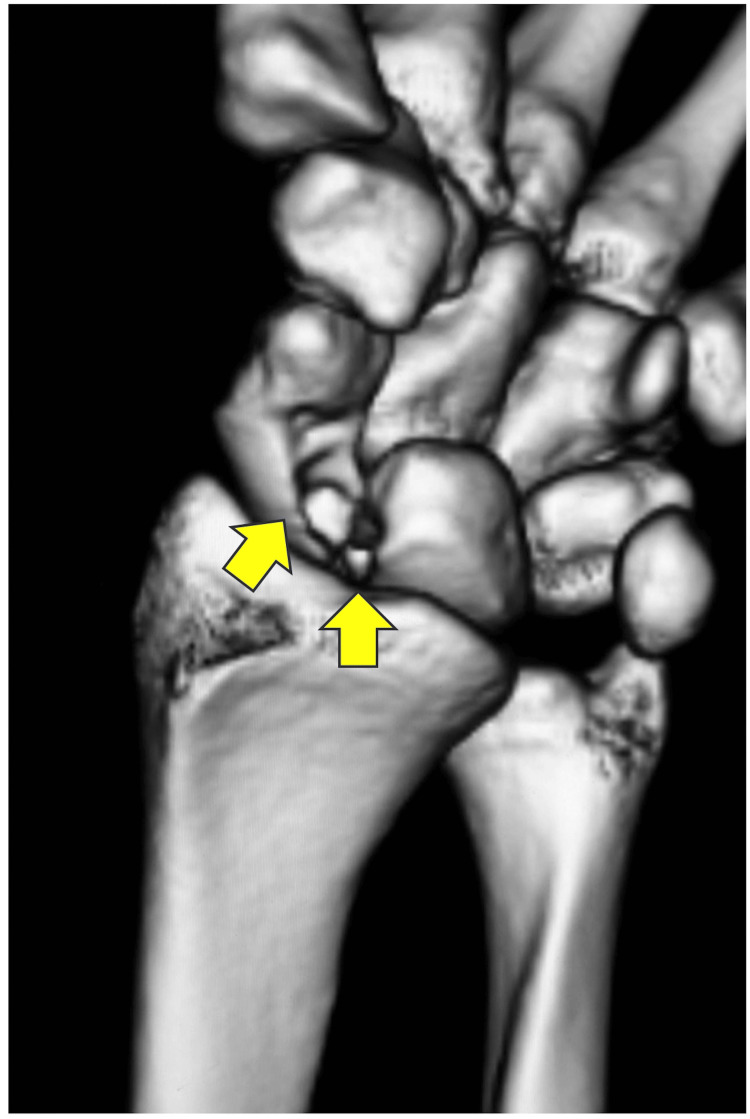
Preoperative three-dimensional computed tomography image of the left wrist (arrows: bony defects).
